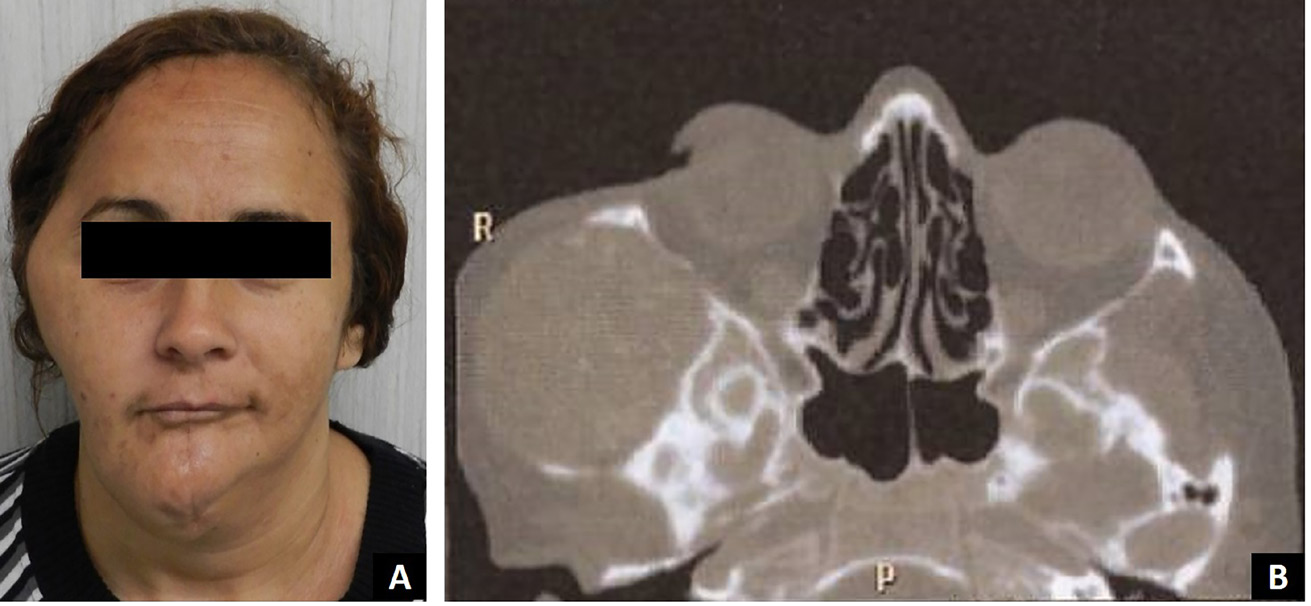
(A) Extra-oral examination: Firm well-circumscribed swelling in right infratemporal region measuring about 7 cm on its longest axis and surgical scar in the middle mandibular region. (B) Axial CT scan showing an expansile solid lesion with internal calcification in the right infratemporal fossa.
